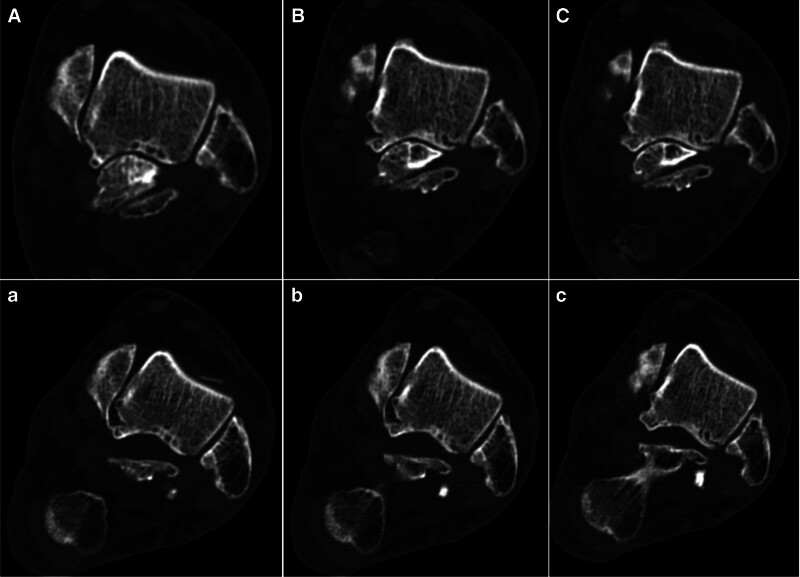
(A–C) Preoperative axial CT scan images show that the diaphyseal radius accounts for about 1/4. (a–c) Postoperative axial CT scan images show that the bipartite talus has been basically resected. CT = computed tomography.
